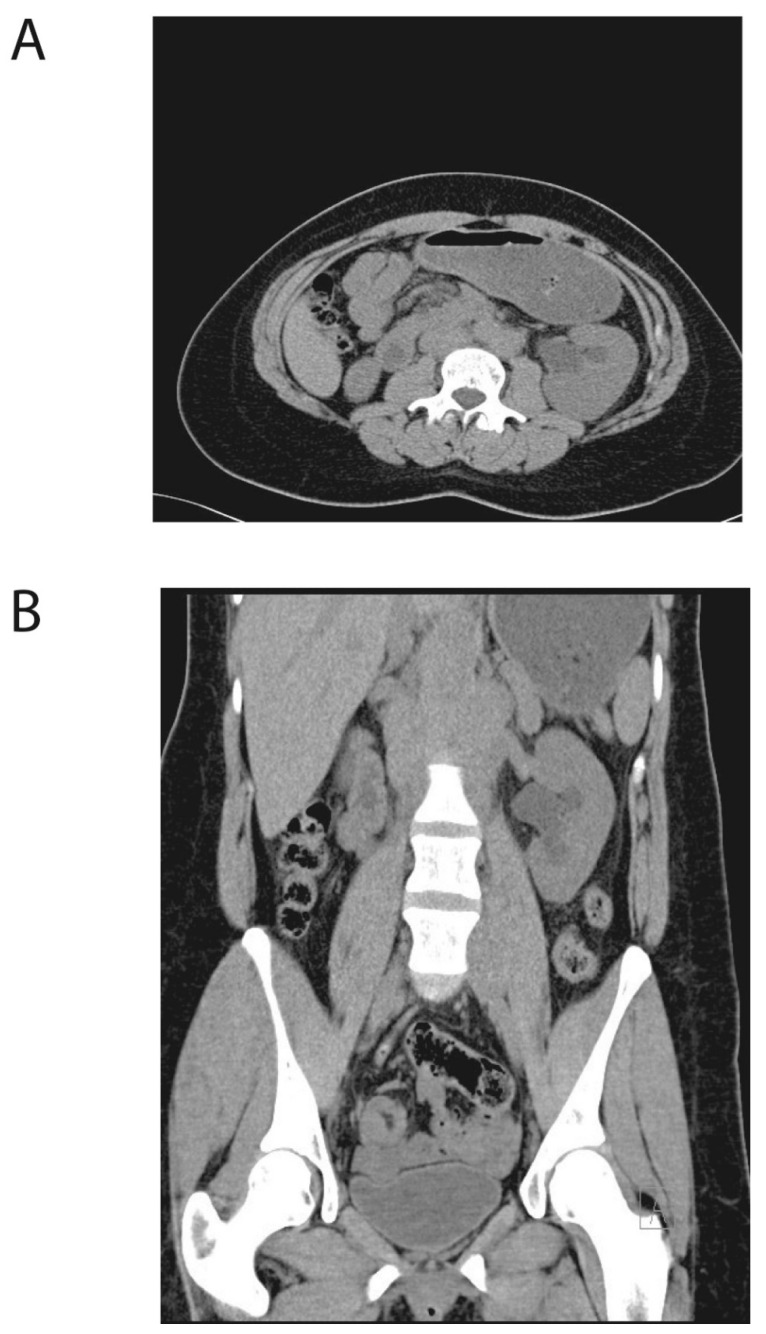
Initial computed tomography (CT) scan of the urinary tract. (A) Transversal plan and (B) frontal plan. The initial CT of the patient’s abdomen, revealing normal kidney parenchyma. The renal pelvis of the left kidney shows dilation compared to right side. The left ureter is dilated and S-shaped near the renal pelvis.
